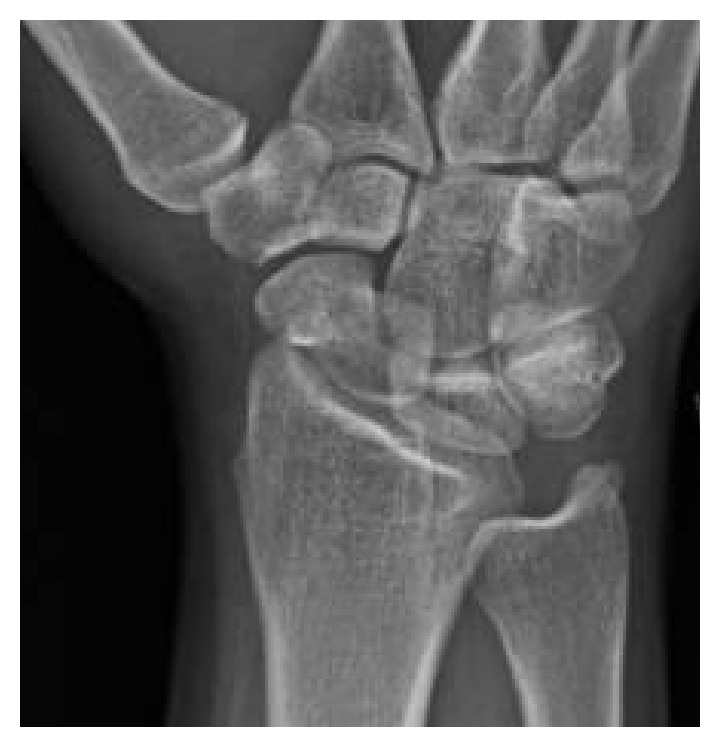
An oblique radiographic view obtained 6 weeks after presentation with no radiographic abnormality of the scaphoid.
